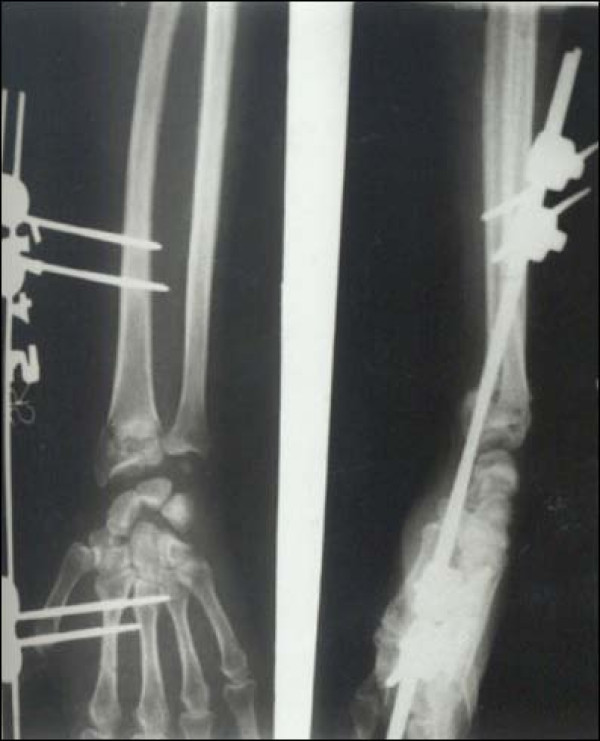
Case 2 Immediate Postoperative Radiograph Anteroposterior and Lateral view.
